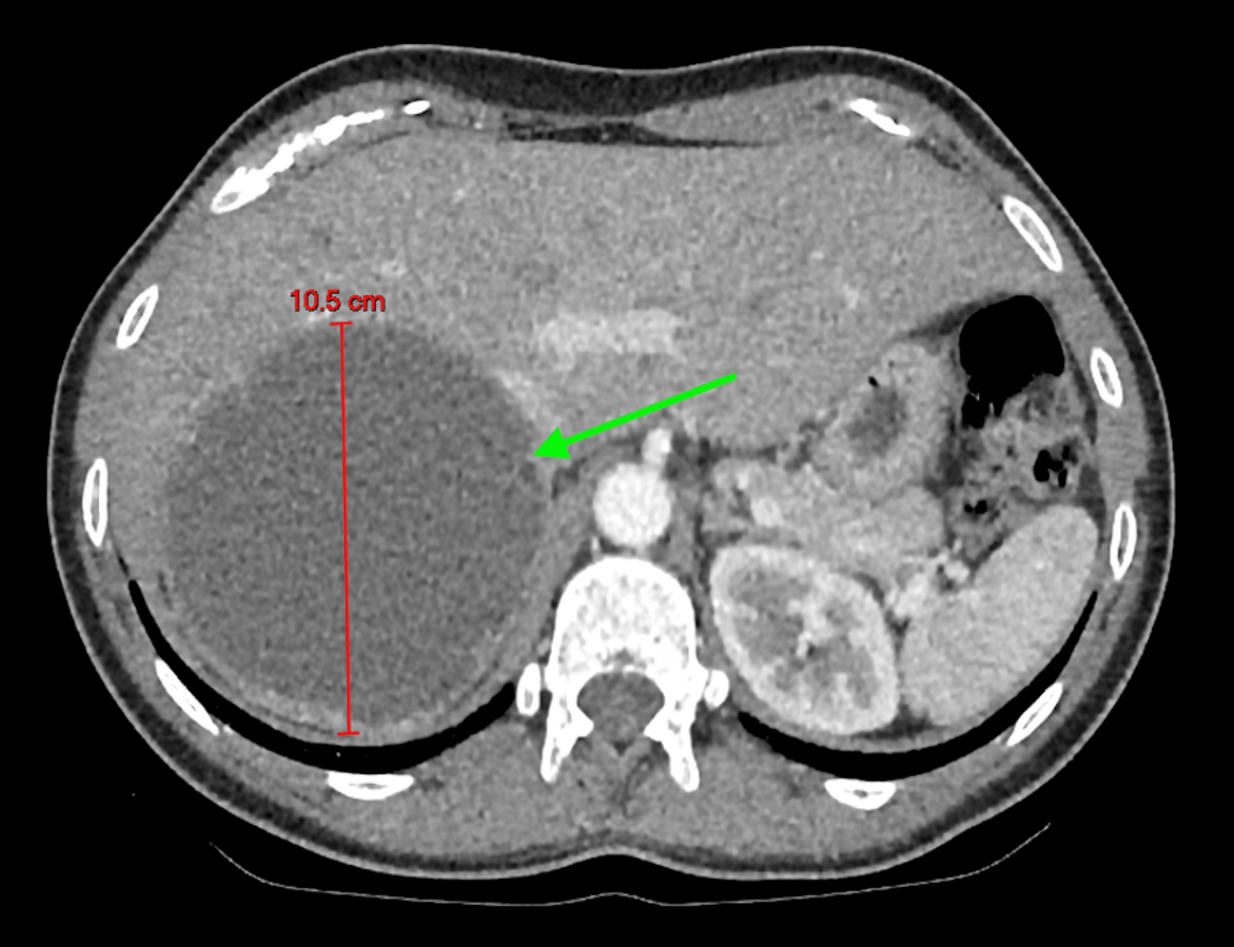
Contrast-enhanced CT of the abdomen revealing a 10.5 cm cystic mass in the right upper quadrant (green arrow), displacing the right kidney and indenting the liver.
